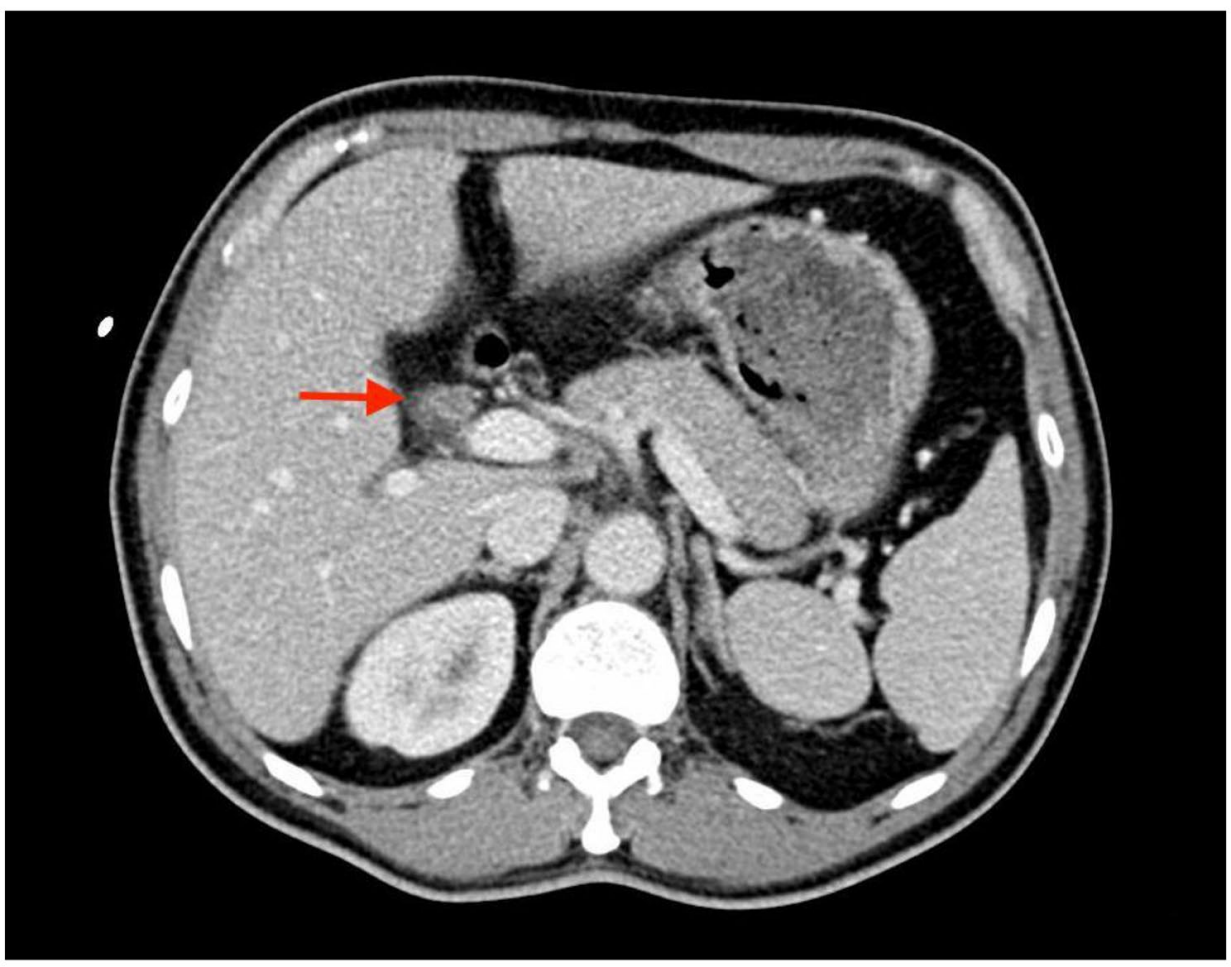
Portal-venous phase CT shows dilation of the bile ducts and an enhanced mass with central hypoattenuation and hyperattenuating rim
